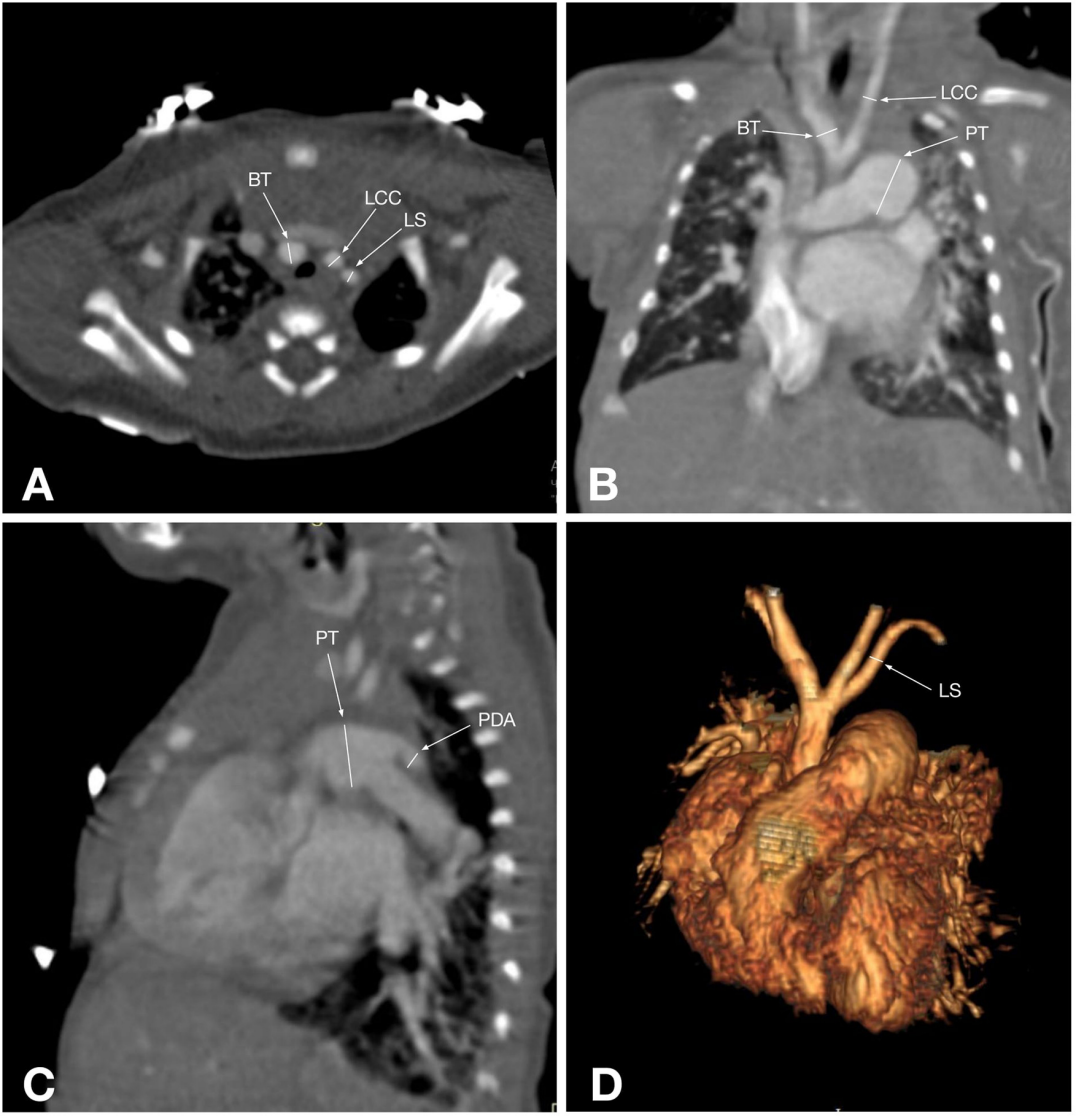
CT angiographic multiplanar images of a 2-day-old patient with type B IAA: (A) axial plane shows the ascending aorta (AAo), followed by the patent ductus arteriosus (PDA), which connects the dilated pulmonary trunk (PT) and the descending aorta (DAo); (B) coronal plane shows the ascending aorta (AAo) and its branch brachiocephalic trunk (BT), next to which are the superior vena cava (SVC) and the enlarged pulmonary trunk (red arrow); (C) Maximum intensity projection of the reformatted sagittal aorta image shows an interrupted aortic arch just distal to the left subclavian artery origin (LS) and the pulmonary trunk (PT), which is connected to the descending aorta via the patent ductus arteriosus (PDA); (D) 3D reconstruction shows an interrupted aortic arch after the right common carotid artery (RCC) arises, and the left subclavian artery (LS) originates from the descending aorta, corresponding to type B IAA.
